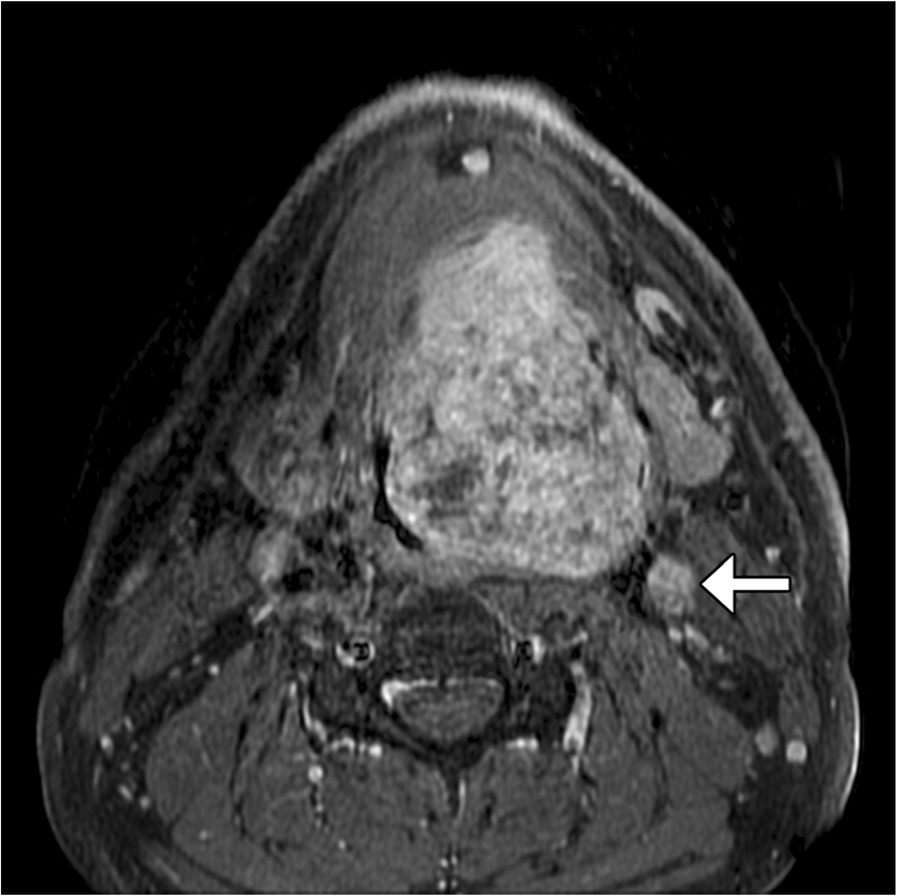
Polymorphous adenocarcinoma (PAC). Axial fat-suppressed T2-weighted MRI shows a bulky heterogeneous mass involving the oral cavity and tongue base with associated left level 2 metastatic lymphadenopathy (arrow)
